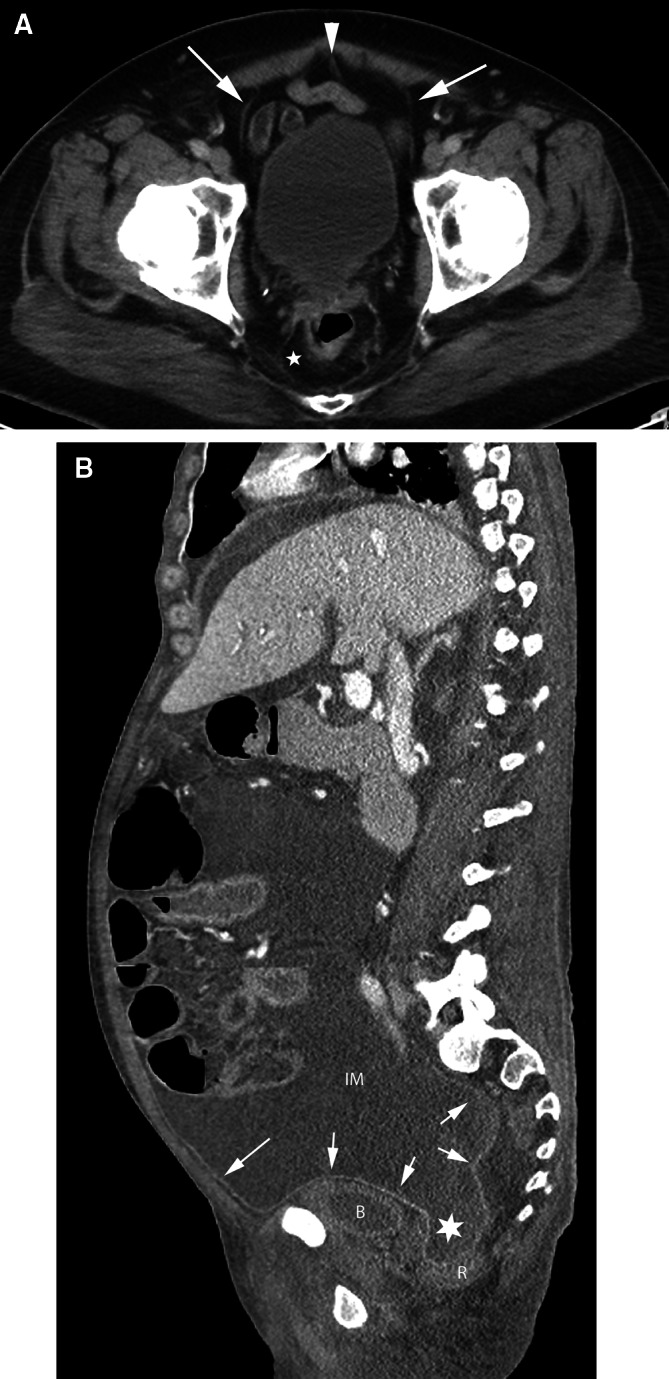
Pelvic spaces. A axial CT image of the pelvis. The urachus (*arrowhead*) and obliterated umbilical arteries (*arrows*) form the median umbilical ligament and the medial umbilical folds, respectively, and are encased within the umbilicovesical fascia forming the perivesical space. The prevesical space is anterior and lateral to the bladder. The perirectal space (*asterisk*) surrounds the rectum. B Sagittal CT image shows the peritoneum (*arrows*) along the anterior abdominal wall and reflecting over the bladder and rectum. Peritoneal fluid in the inframesocolic compartment (IM) communicates with the rectovesical recess (*asterisk*). The bladder is inferiorly displaced by the peritoneal fluid. *B* bladder, *R* rectum.
